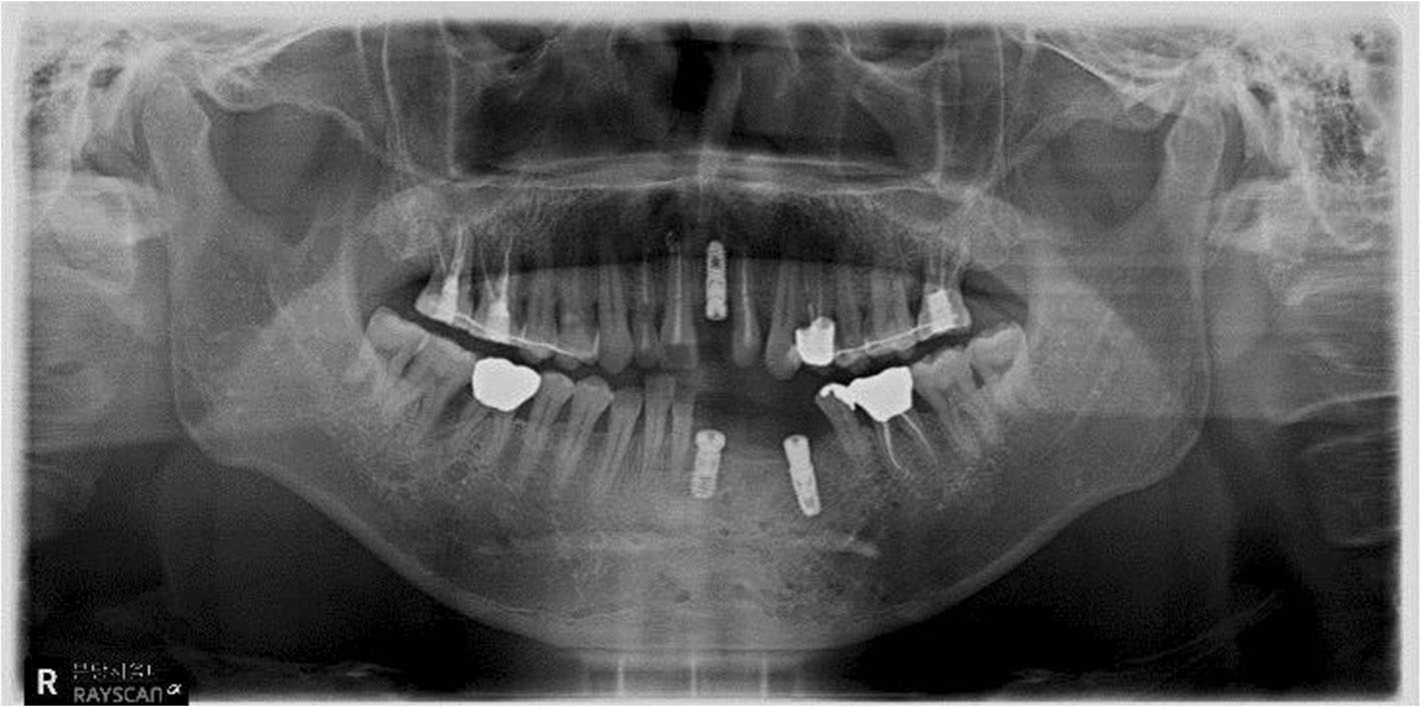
Panoramic radiograph after implant placement
